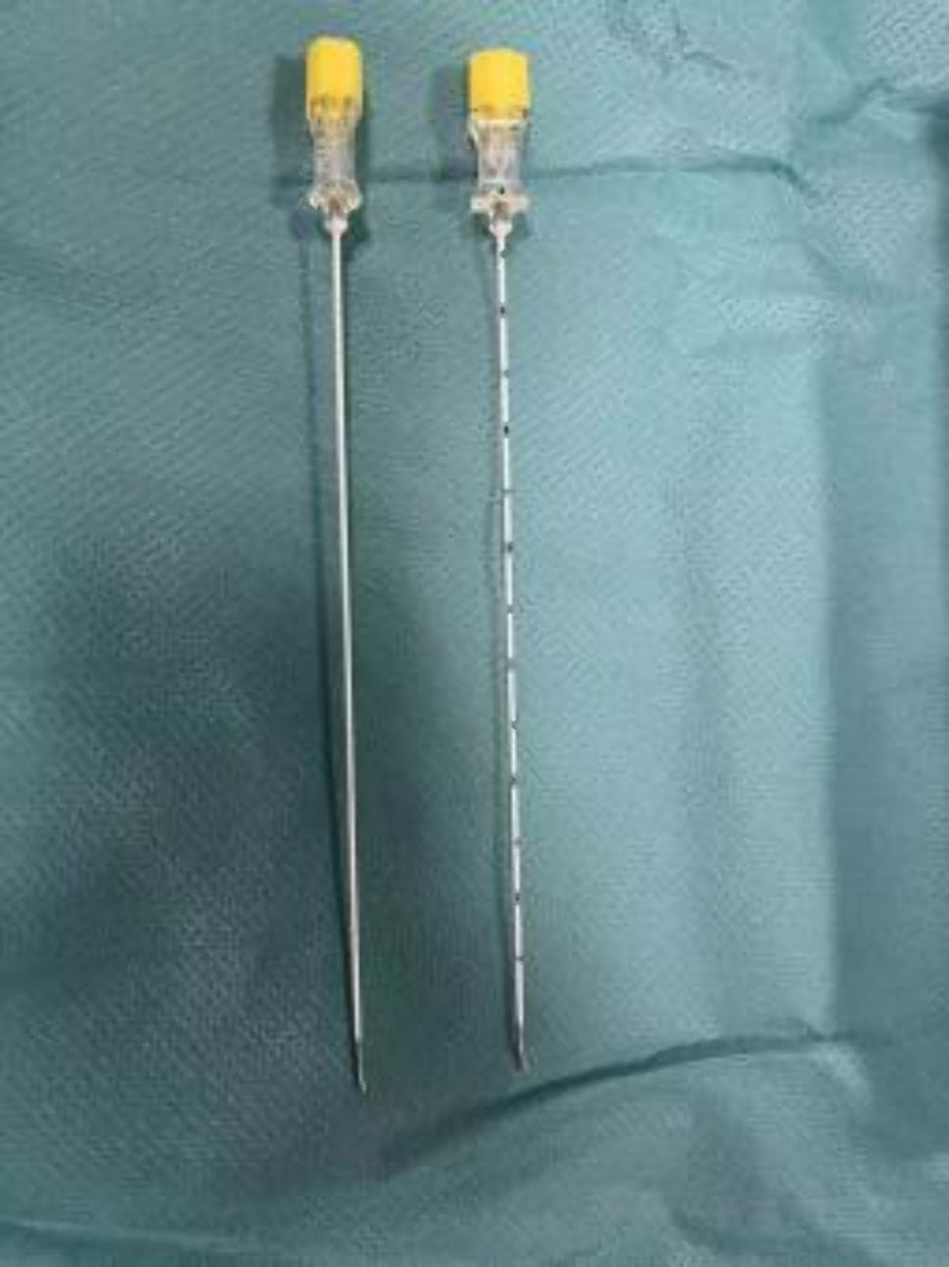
Specially made blunt puncture needleRadiofrequency thermocoagulation of lumbar sympathetic nerveLumbar sympathetic nerve radio-frequency (RF) technology is relatively mature. Taking the L3 sympathetic nerve RF as an example, the surgical procedure was as follows: The patient was placed in a prone position, the third lumbar vertebra was scanned, and the layer thickness was set to 2 mm. The appropriate injection path, angle, and depth were chosen to avoid nerves, blood vessels, and organs. Metal marks are made on the skin surface of the human vertebral body during CT scanning. The skin was disinfected, covered, and administered local anesthesia. The puncture was performed slowly using a radiofrequency electrode trocar. The CT scan confirmed that the needle tip reached the target position, namely the anterolateral edge of the L3 vertebral body and the front of the psoas major muscle (Fig. 8). If the patient does not have radiating pain in the lower extremity or groin area and has a sense of swelling in the waist, there is no sensory nerve in the damaged area. After motor nerve stimulation at 1 V and 2 Hz, there were no convulsions in the muscles of the lower extremities or buttocks and there were no motor nerves in the damaged area. The puncture needle is gradually warmed from 42 ℃ to 95 ℃ for 300 s of thermocoagulation treatment, after which the needle is withdrawn. The patient was returned to the ward when vital signs were stable.
